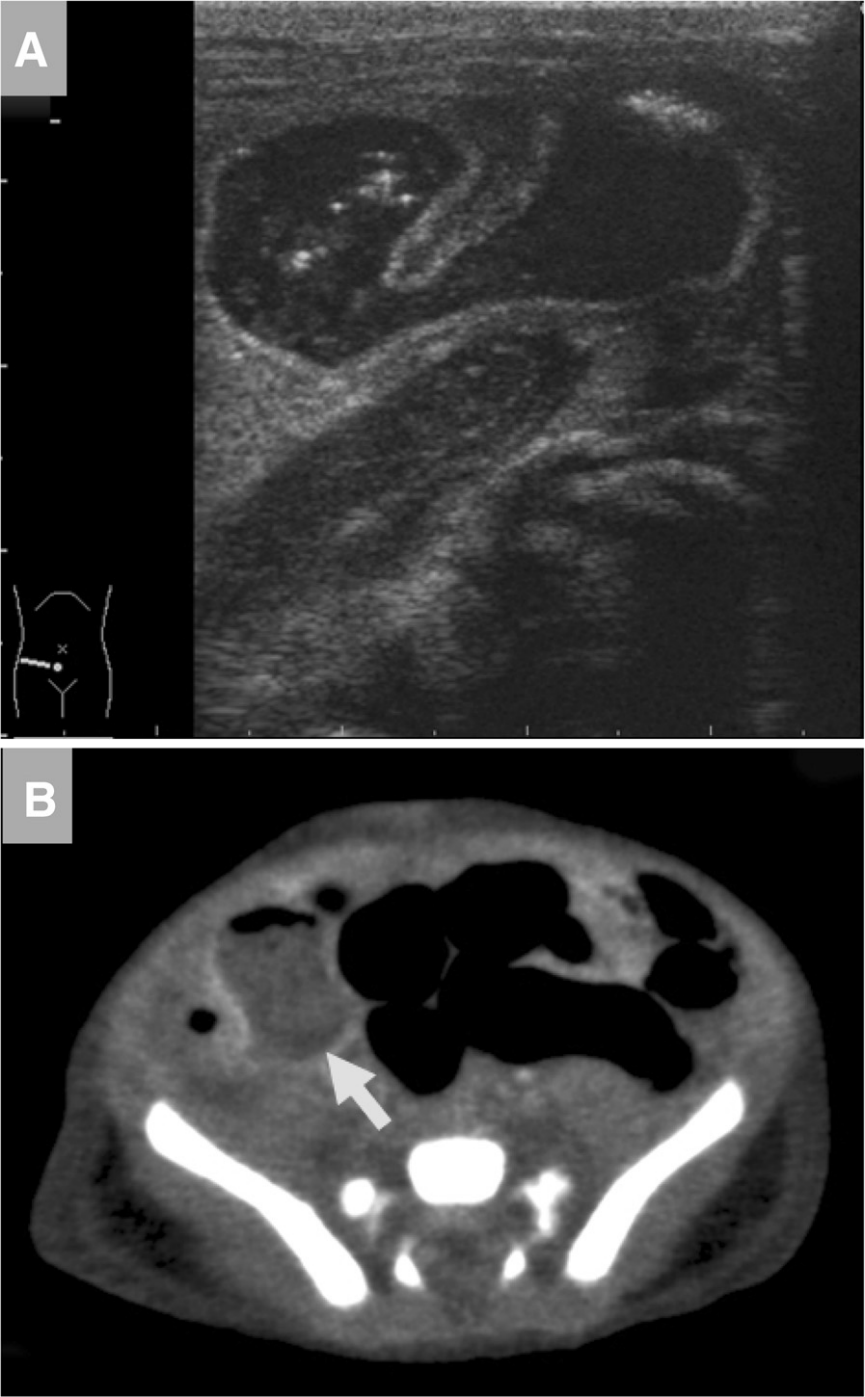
Ultrasonography and computed tomography images.A) Ultrasonography shows an intra-abdominal cyst with a double layer surrounding sign in the right lower abdomen. B) Computed tomography shows a cyst with an enhanced wall (Arrow).
